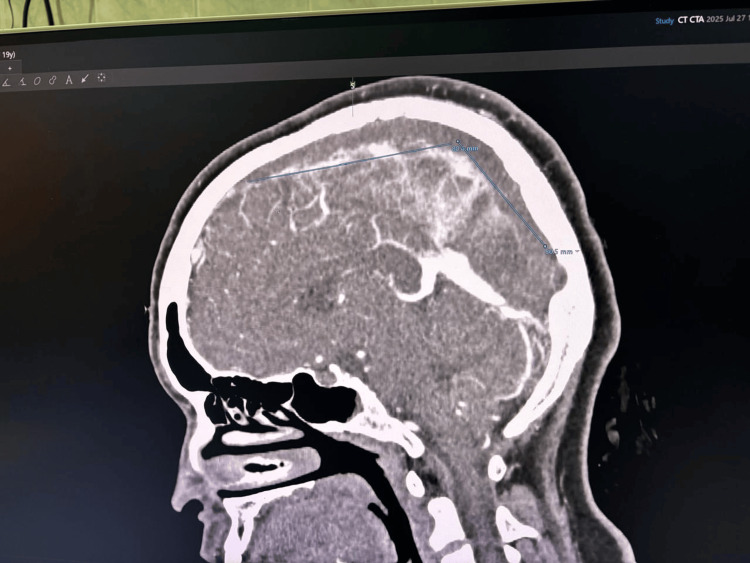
Extensive superior sagittal sinus thrombosis in CT venogram
